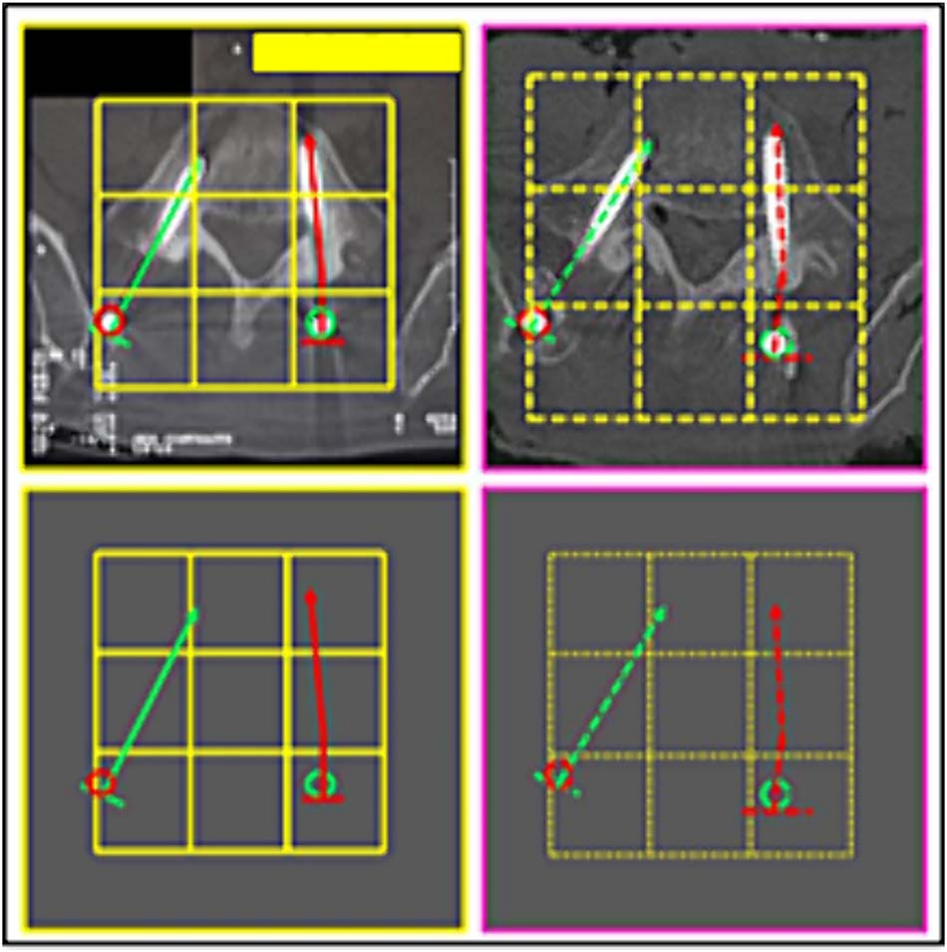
L5 vertebral body. Upper panels, comparison between the axial slices of the antemortem (left) and postmortem (right) CT scans. Lower panels, respective concordant templates.
